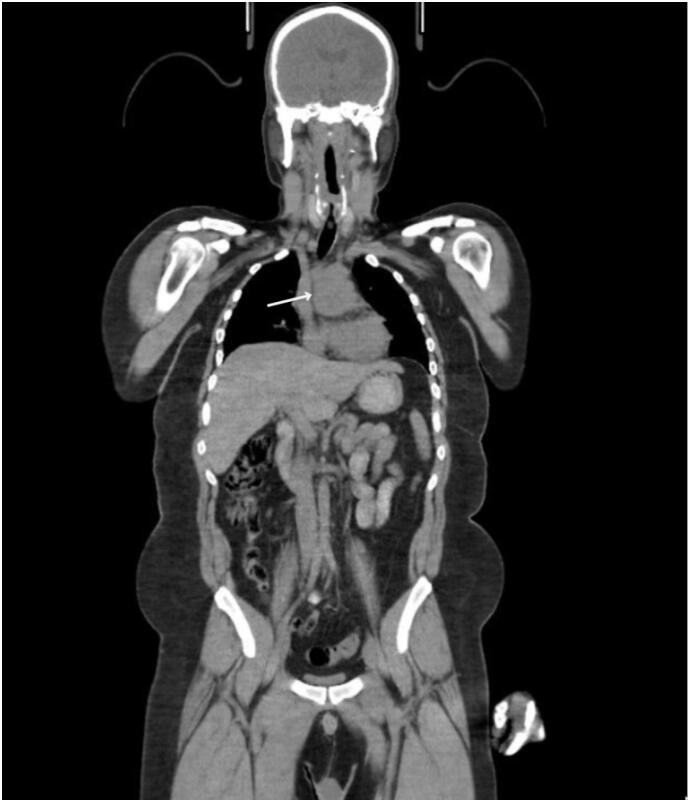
Coronal view of CT Chest showing mass occupying aortopulmonary space.
